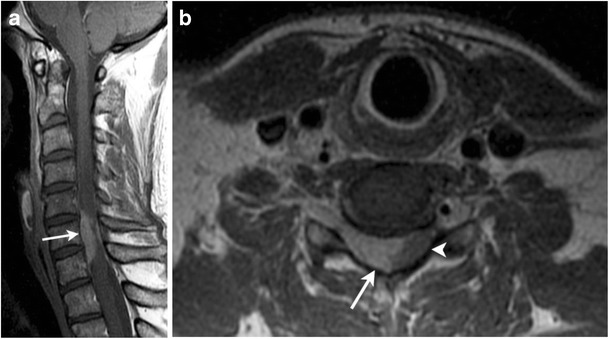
A 36-year-old male with newly diagnosed nodular sclerosing HL presenting with numbness in his right hand. a Sagittal contrast-enhanced T1-weighted image of the cervical spine demonstrates enhancing epidural soft tissue (*arrow*) at the C4 to C6 vertebral levels. b Axial contrast-enhanced T1-weighted image demonstrates epidural enhancing soft tissue (*arrow*) with significant compression and mass effect on the cervical spinal cord (*arrowhead*)
